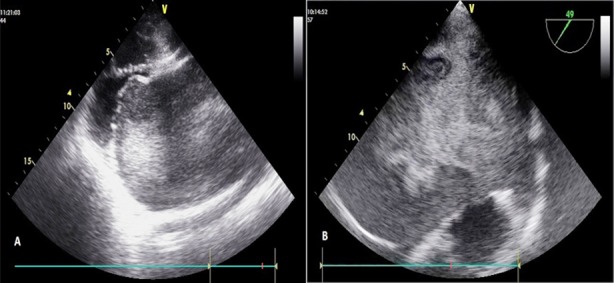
A. Transthoracic echocardiography left parasternal long axis view showing features of severe mitral stenosis, dilated left atrium and dense, spontaneous echo contrast. B. Transesophageal echocardiography midesophageal view at 49° showing dilated left atrium, dense, spontaneous echo contrast, and appendage free of definite thrombus.
